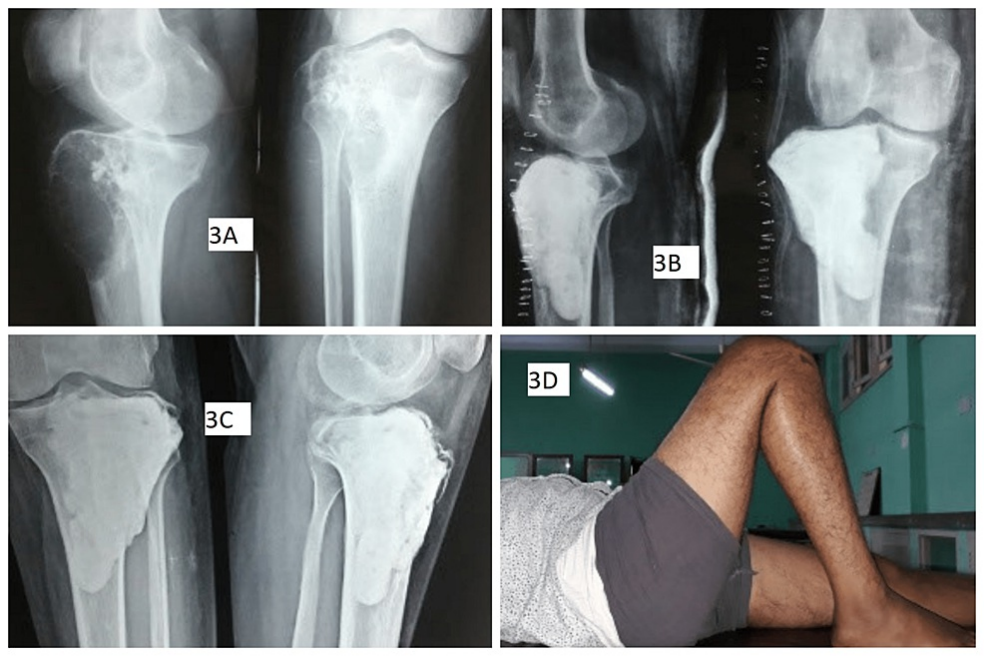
Recurrent GCT of proximal tibia in a 24-year-old male who was treated with extended curettage and bone cementing: (A) Preoperative radiograph showing recurrent GCT in proximal tibia; (B) Postoperative radiograph showing extended curettage with bone cementing; (C) Follow-up radiograph after six months; (D) Functional outcome GCT: giant cell tumor
